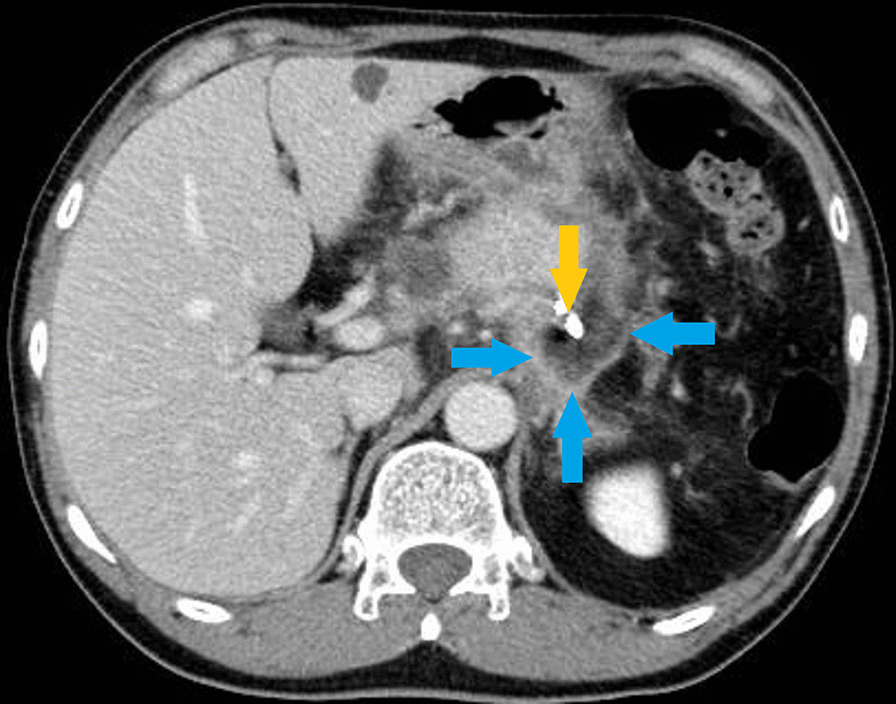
Contrast-enhanced computed tomography examination on day 134 after surgery. The yellow and blue arrows indicate the drain and the abscess cavity, respectively
